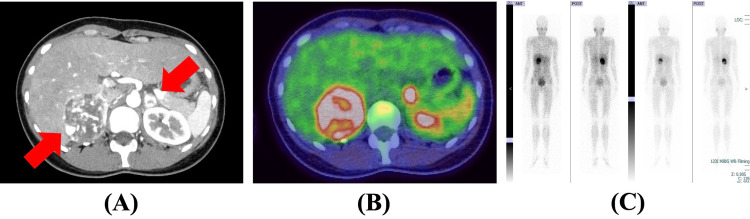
Imaging finding of bilateral adrenal tumors (A): A contrast-enhanced CT image showing bilateral adrenal tumors (arrow). (B): A fluorodeoxyglucose positron emission tomography image showing abnormal uptake on bilateral adrenal tumors. (C): ^123^I-metaiodobenzylguanidine scintigraphy showing increased uptake in the right adrenal gland.
